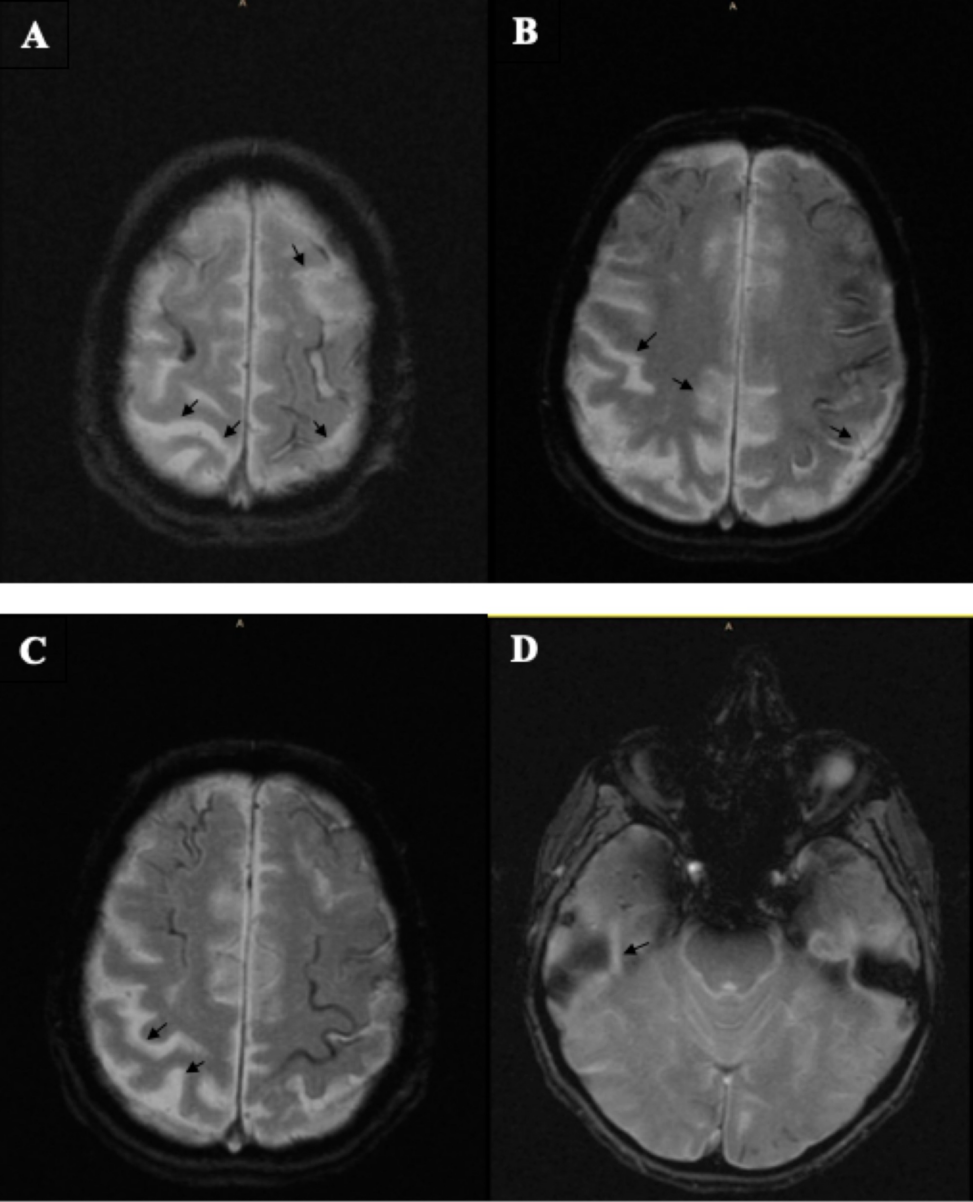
Detection of cortical superficial siderosis on MRI Distribution of cSS is supratentorial with no intraparenchymal involvement as is seen in ICH. A. cSS; B. bilateral temporal; C. left temporal; D. right temporal and left occipital lobes cSS, cortical superficial siderosis; ICH, intracerebral hemorrhage
